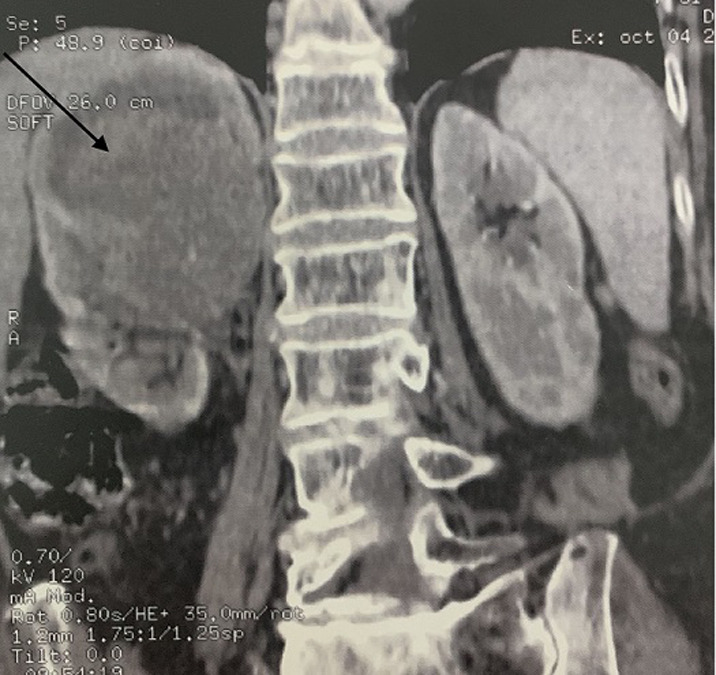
abdominal computed tomography scan showing a mass in the right kidney 86 x 76 x 68 mm
